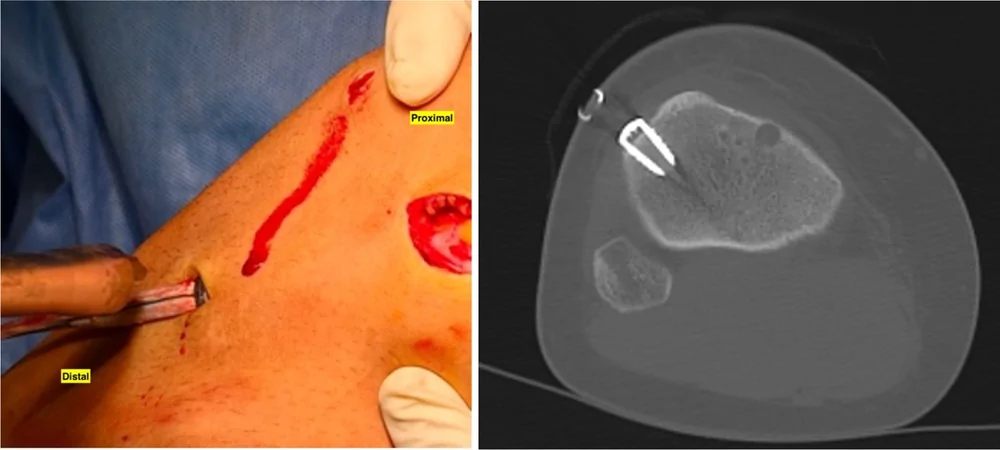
(a) Final position of the tibial bone staple following fixation. (b) Axial CT view demonstrating ideal placement of the tibial staple seated against the lateral tibial cortex.
